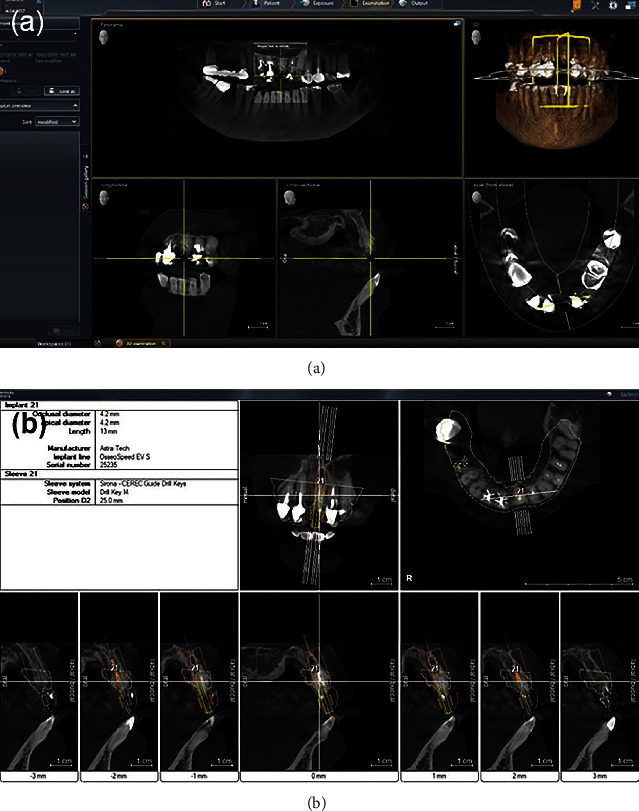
Radiograph for the diagnosis and treatment planning (a, b).
